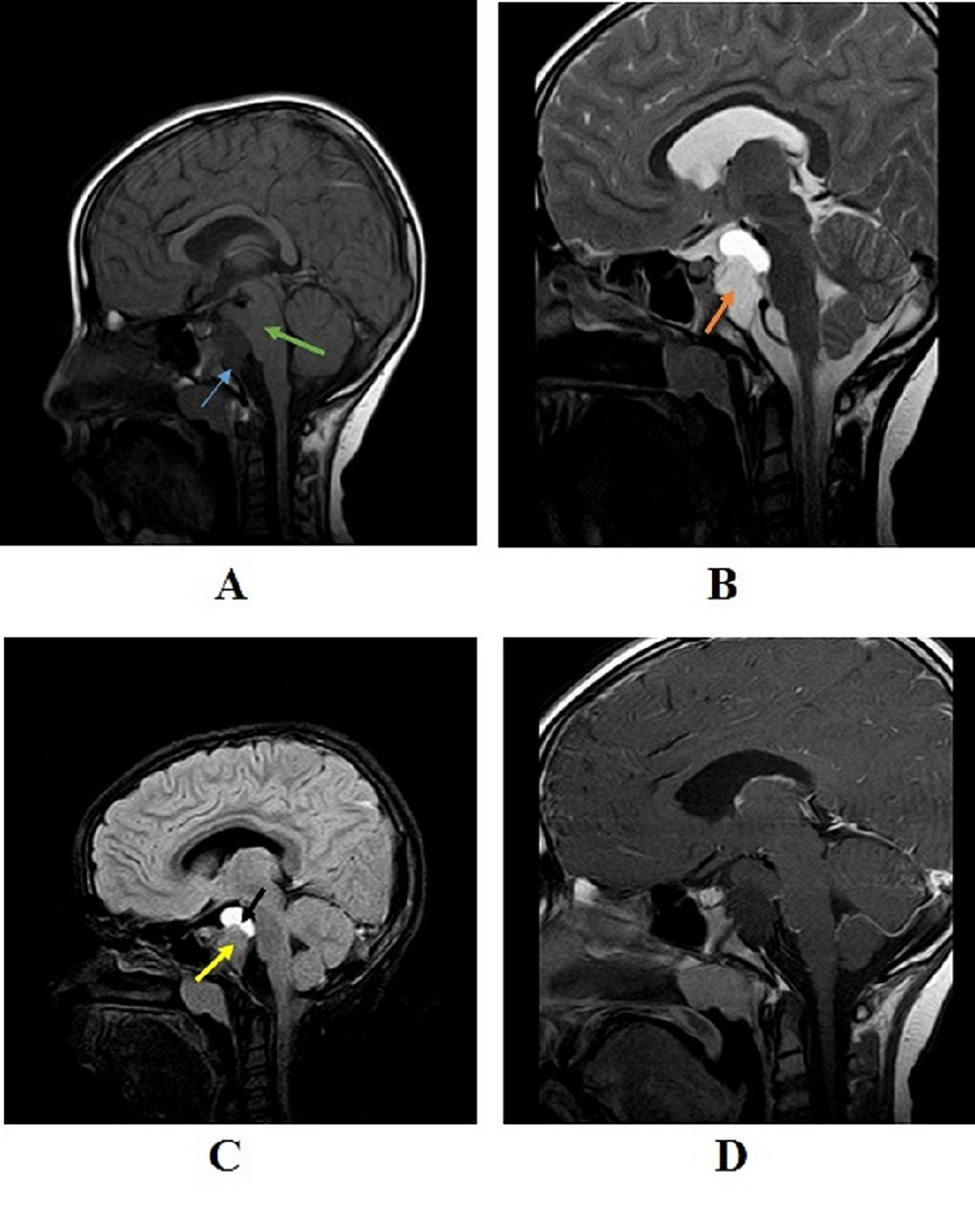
MRI scans of the patient MRI scans of the patient with (A) sagittal T1WI showing bilobed solid and cystic intradural lesion in the prepontine cistern (blue arrow), and cystic component showing isointense signal (green arrow); (B) sagittal T2WI showing hyperintense signal of the solid and cystic components (orange arrow); (C) sagittal fluid-attenuated inversion recovery (FLAIR) showing isointense signal of the solid (yellow arrow) and hyperintense signal of the cystic component (black arrow); and (D) sagittal T1 post-gadolinium showing no enhancement in the lesion.
